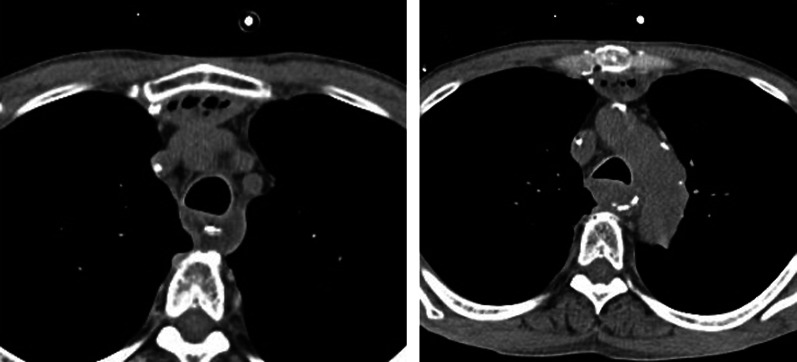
Findings of CT after esophageal drainage. The CT scan showed that the mucocele was still absent 2 months after the surgery
